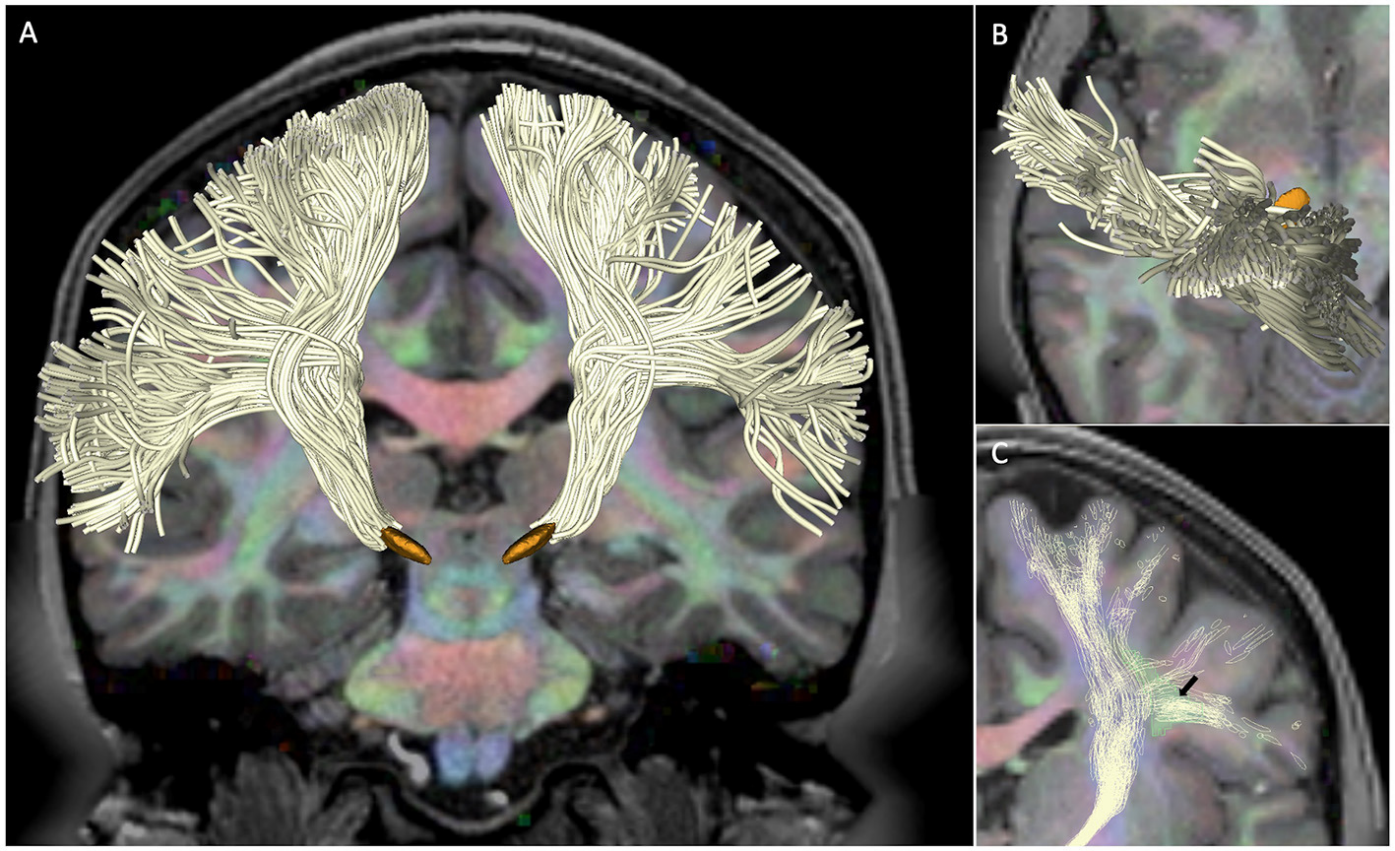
Hyperdirect pathway in subject 100307. The figure shows the tractography reconstruction of hyperdirect pathway fibers projecting from the primary motor cortex to the subthalamic nucleus. The tracts (white) are displayed on a diffusion encoded color map overlaid on a T1-weighted image with 3D models of the subthalamic nucleus (orange) for anatomical reference. (A) Anterior 3D view of the hyperdirect pathway. (B) Superior 3D view of hyperdirect pathway fibers descending from the primary motor cortex. (C) Anterior 3D view of the crossings of hyperdirect pathway fibers with the superior longitudinal fasciculus. The arrow points at the intersection of lateral projections of the hyperdirect pathway with a cross-section of the superior longitudinal fasciculus (green).
